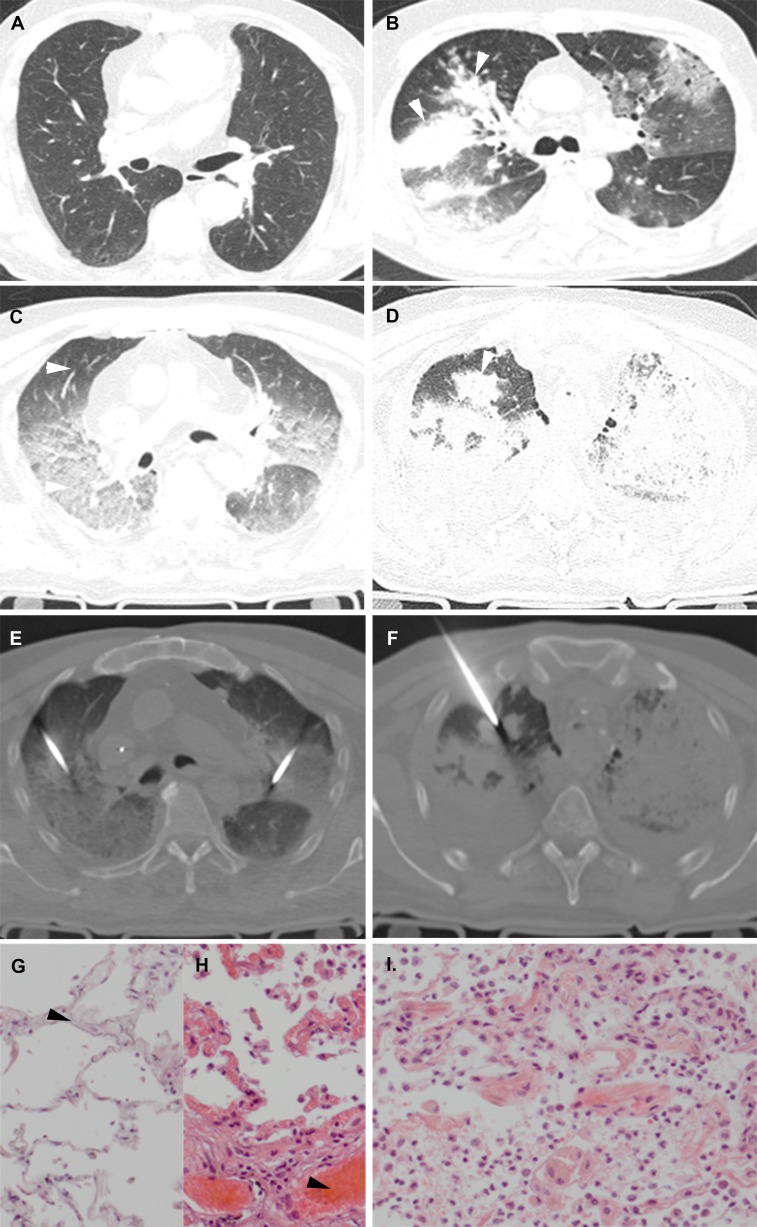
Internal livores of the lungs versus pneumonia. (A/C) antemortem CT (A) and PMCT (C). Normal (A) and internal livores (C) (arrowheads). (B/D) antemortem CT (B) and PMCT (D). Patient with a pneumonia (arrowheads). (E) CT-guided lung biopsies in the same patient as A/C. (F) CT-guided lung biopsy in the same patient as B/D. (G) HE, x100 original magnification. Lung parenchyma non-dependent: capillaries in alveolar walls practically devoid of blood (arrowhead). (H) HE, x100 original magnification. Same patient as G, lung parenchyma dependent, capillaries in alveolar walls congested with blood (arrowhead). (I) HE, x100 original magnification. Same patient as B/D/F, lung parenchyma, resolving pneumonia with thickened alveolar walls with mainly lymphocytic infiltrates, and hyaline membranes and extravasations of erythrocytes in the alveolar spaces.
